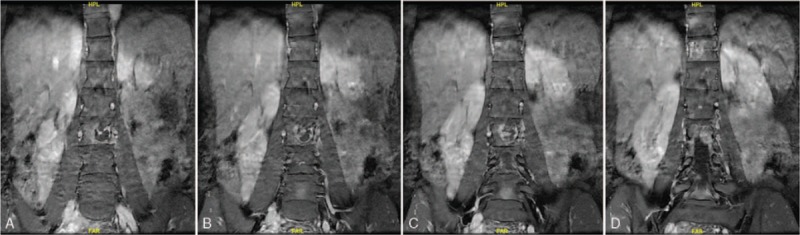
(A–D) Preoperative coronal MRI scan revealing the density of soft tissues and obvious bony destruction in the T12 and L3.
